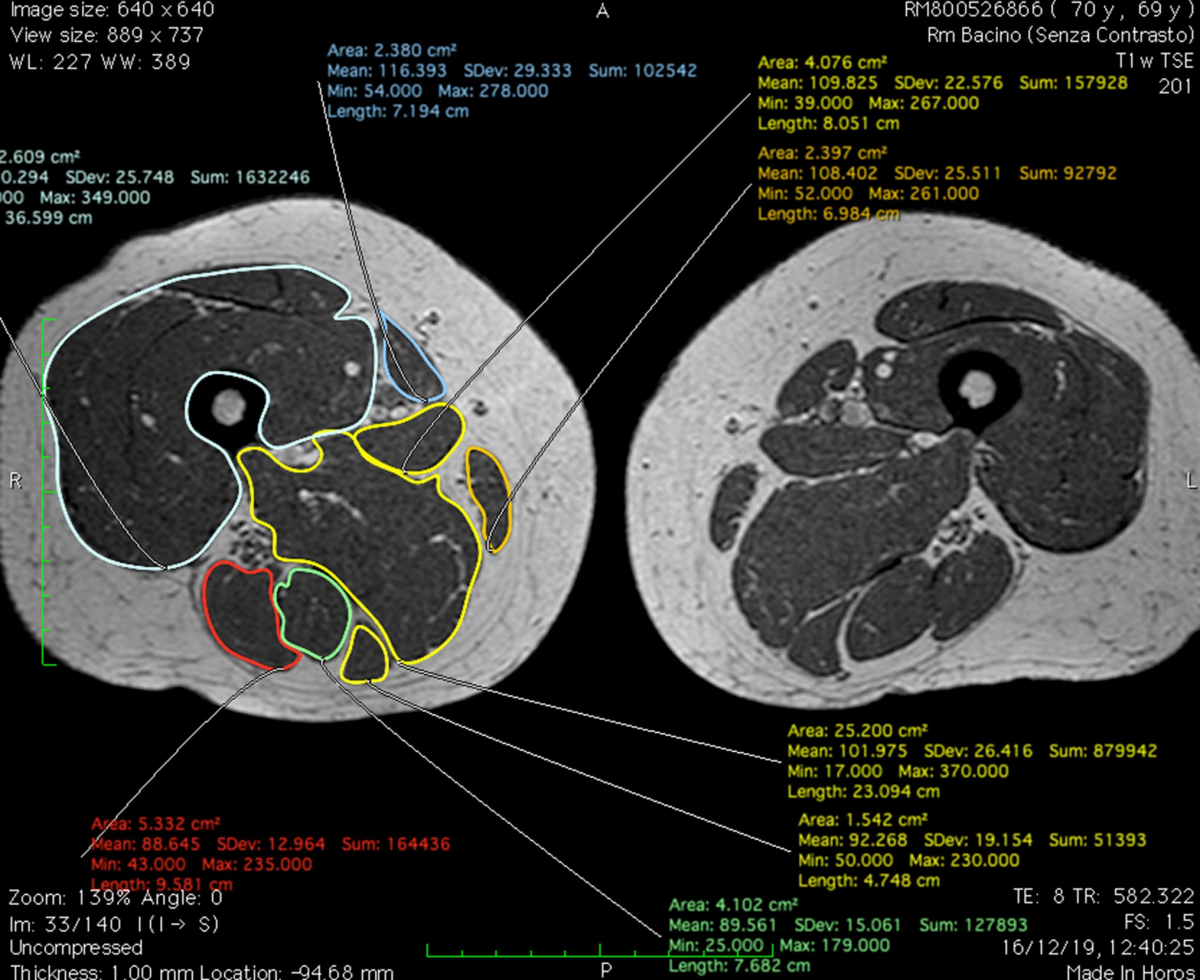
Representative assessment of cross-sectional area on magnetic resonance image of the thigh with rectus femoris, vastus lateralis, vastus intermedius and vastus medialis labeled, using “closed polygon” function (software Horos)
